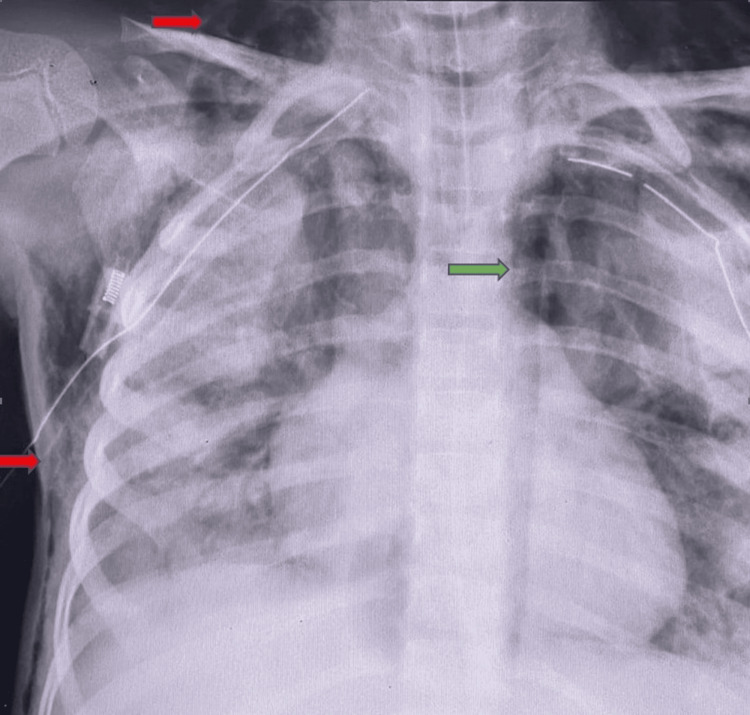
Chest X-ray after complication with air leak syndrome. Chest X-ray showing pneumothorax (green arrow) requiring chest tube insertion and extensive surgical emphysema (red arrow).
